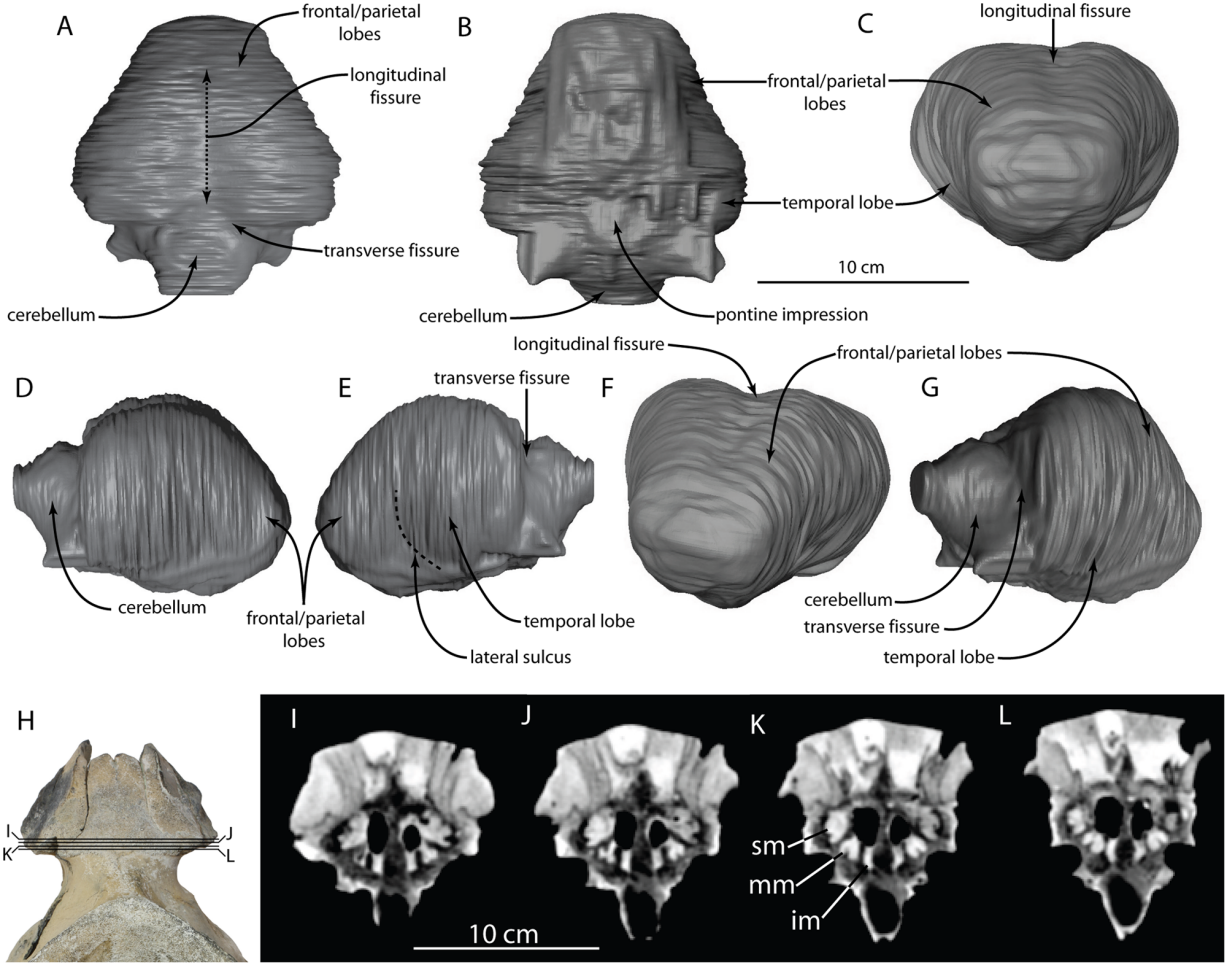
Albertocetus meffordorum* endocast (CCNHM 218) in (A) dorsal, (B) ventral, (C) anterior, (D) right lateral, (E) left lateral, (F) anterodorsolateral, and (G) posterolateral views; (H), photograph of skull showing positions of CT slices; (I-L) CT slices of interorbital region showing ethmoid labyrinth. Abbreviations: IM, Inferior Meatus; MM, Middle Meatus; SM, Superior Meatus.
